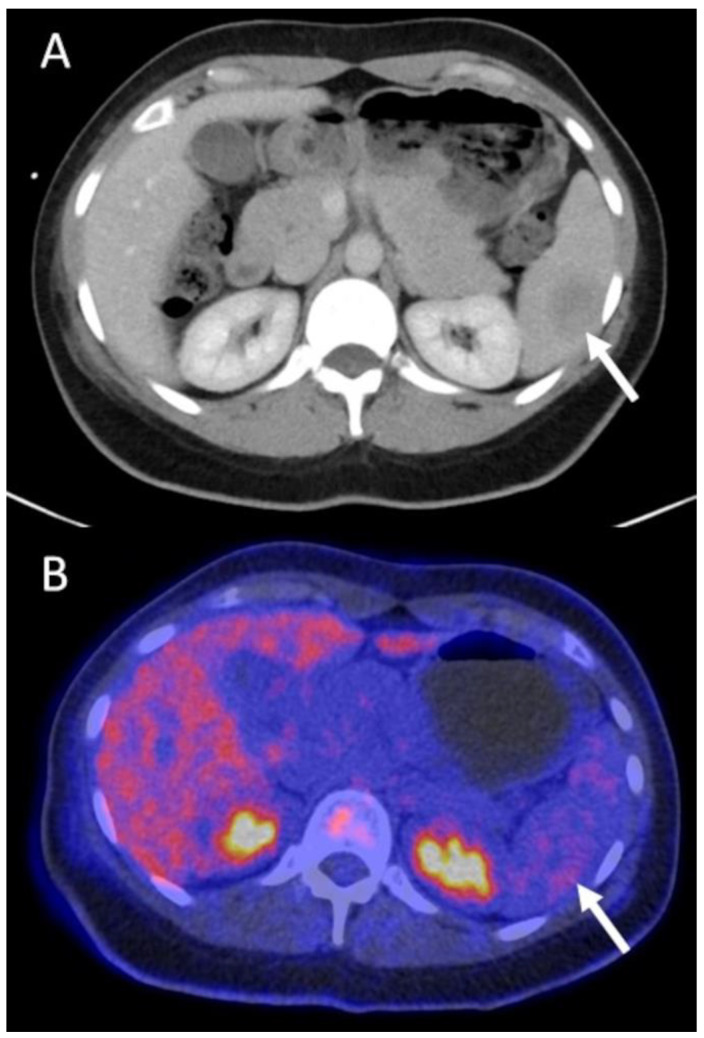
A 37-year old female patient with grade 3 NST of the left breast. Tumor stage pT3, pN1. Initial primary staging CT shows an ill-defined, slightly hypodense splenic lesion, highly suspicious for a distant metastasis (arrow in (A)). Additional FDG PET/CT does not show elevated tracer-uptake, excluding a metastasis, thus ruling out M1 stage and leading to a major change in management (arrow in (B)). NST = invasive carcinoma of no special type.
